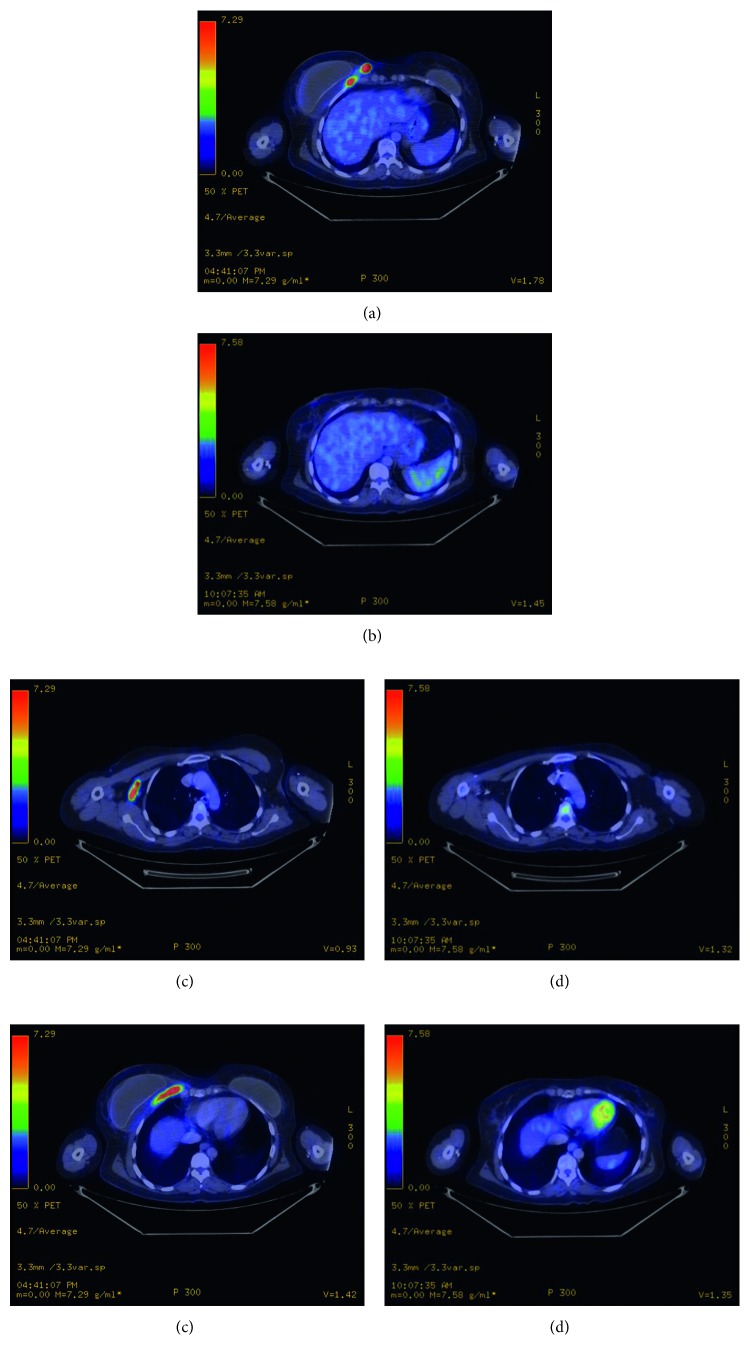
Pretreatment PET/CT staging scan showing right medial breast involvement (a) and restaging PET/CT after surgery and chemotherapy showing complete resolution of disease in the breast (b), pretreatment PET/CT showing right lymph node involvement (c) and posttreatment decrease in size of the nodes (d), and pretreatment right chest wall involvement (e) and posttreatment resolution of disease in chest wall (f).
